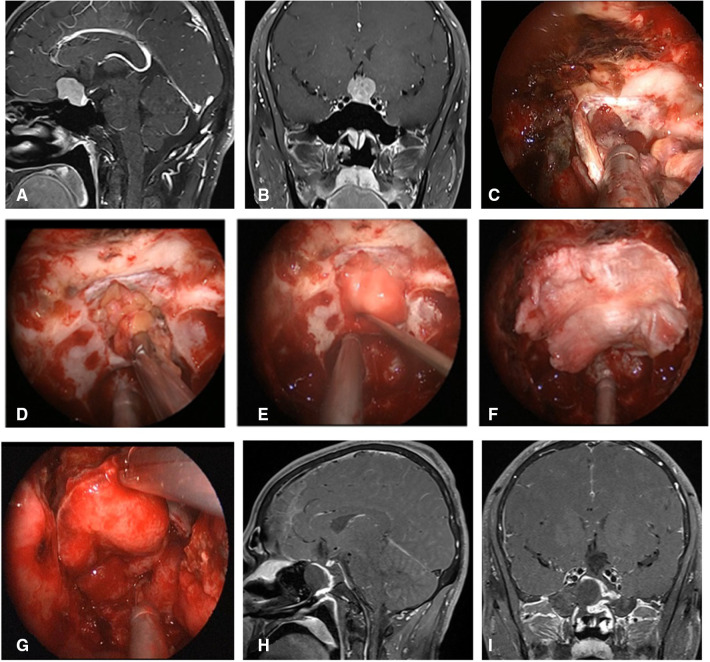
Repair process of patient with insufficient embedded fat. (A,B) MRI enhanced in sellar region before operation and was considered pituitary adenoma. (C) Uncover the VP-NSF to see the subdural leakage. (D) Subdural fat packing. (E) Artificial dura mater embedded between cellulite and dura mate. (F) Cover fascia lata on the artificial inlay dura (arrow). (E) Cover the VP-NSF on the fascia lata. (H,I) Sagittal and coronal enhanced MRI in sellar region after operation, and postoperative pathology showed pituitary adenoma. VP-NSF, vascularized pedicle nasoseptal flap.
